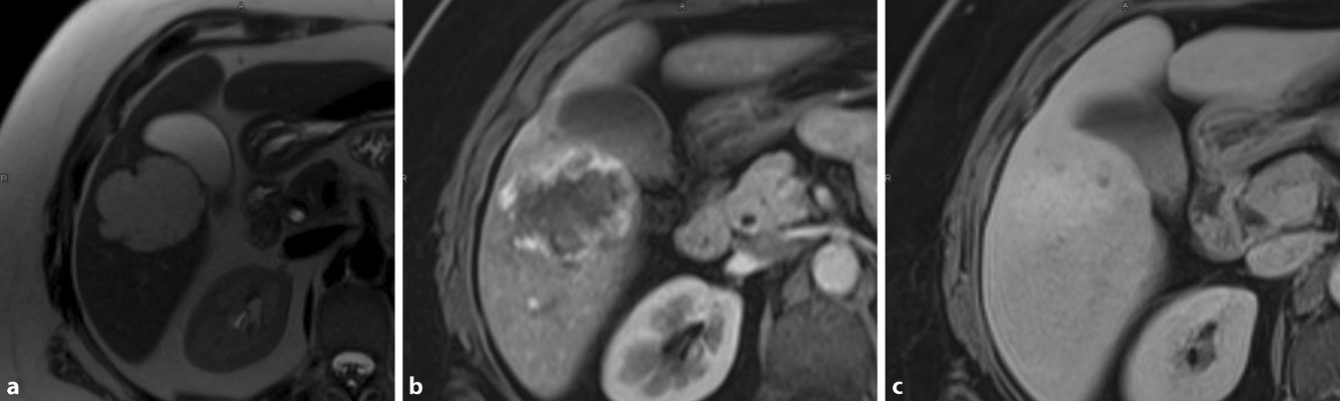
Haemangioma in segment V: axial T2-weighted (a), arterial phase (b) and late phase approximately 2 min after the i. v. injection of contrast agent (c). Typical hyperintense signal in the T2-weighted images and the peripheral enhancement in the arterial phase with nearly isointense presentation in the late phase
